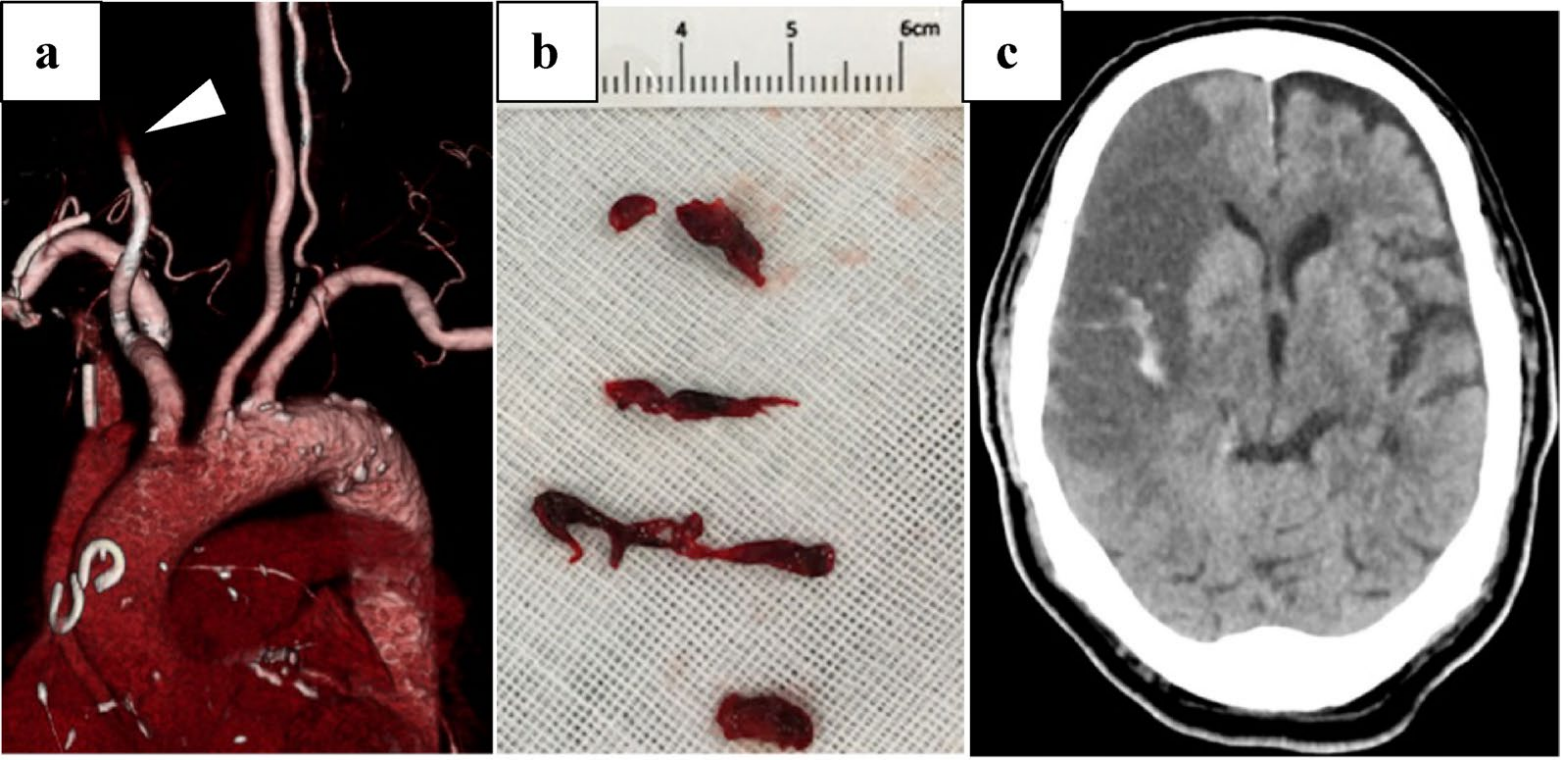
a: Right carotid embolization on computed tomography. b: Thrombus from the right carotid artery. c: Hemorrhagic brain infarction on computed tomography
